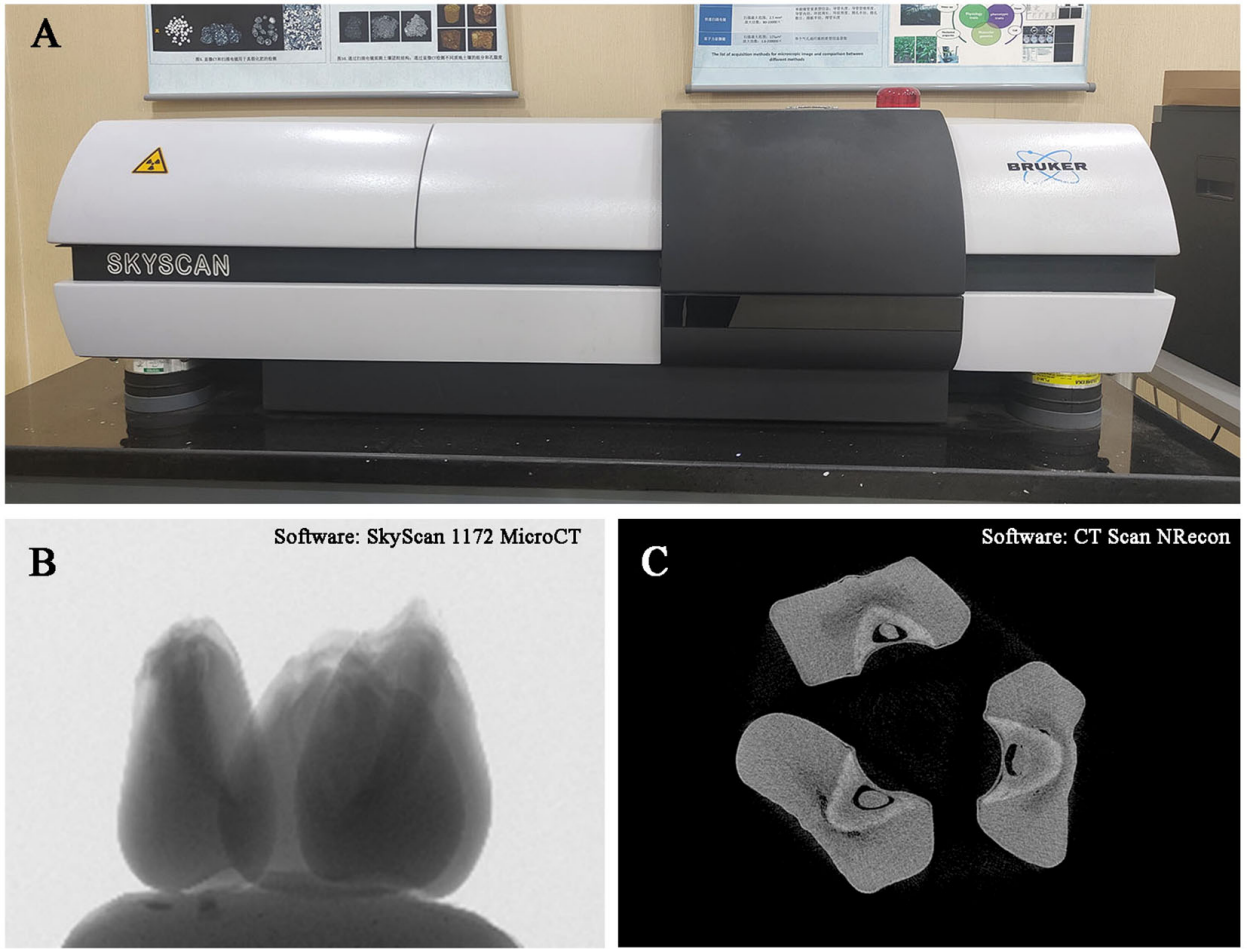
CT scanning instrument (A) and kernel reconstruction (B, C).
